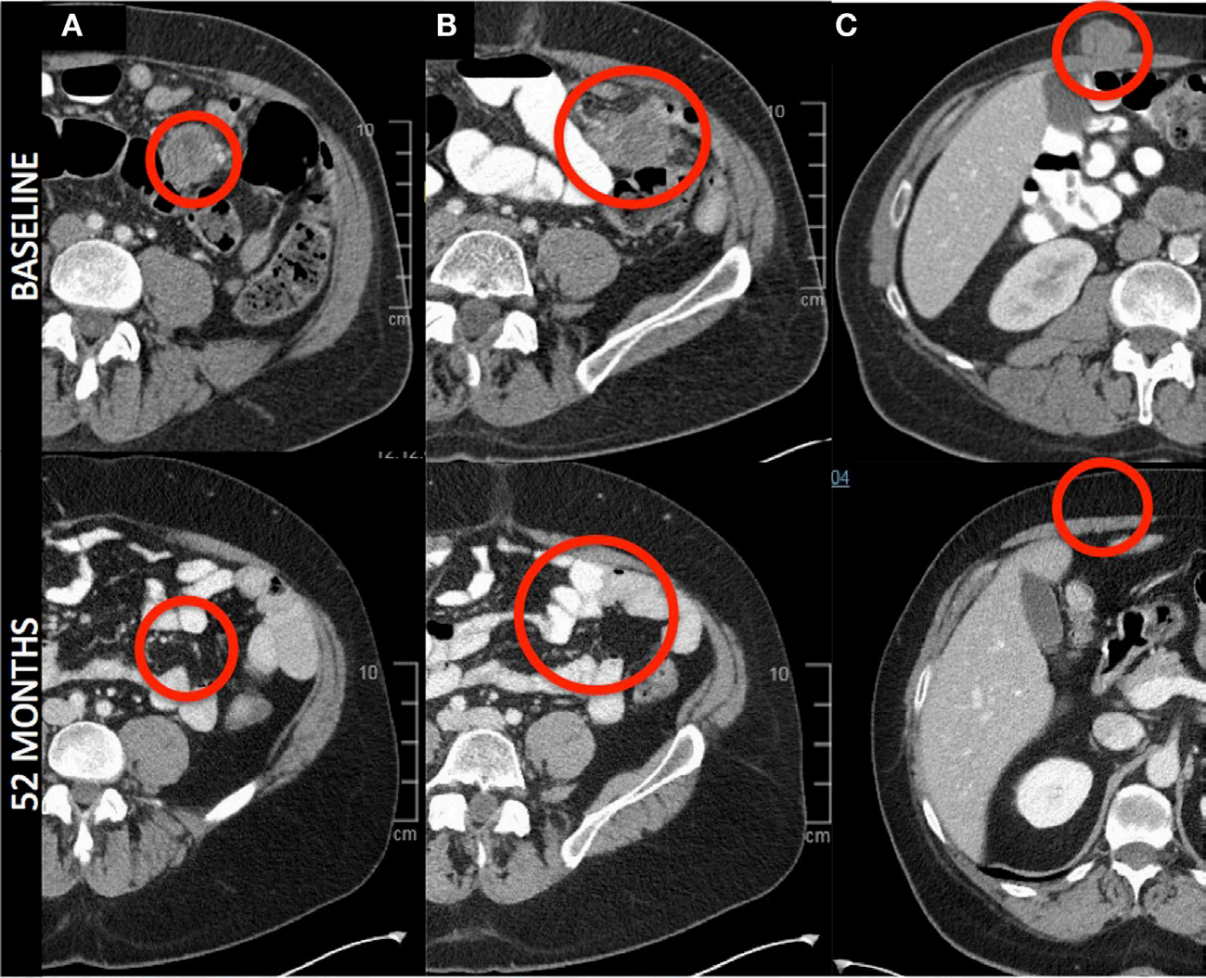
Radiographic response—axial images from a patient with a complete response. Images denote complete resolution of disease in the mesentery (A,B), and abdominal wall (C). All other lesions (liver and posterior abdominal wall, not pictured) completely regressed.
